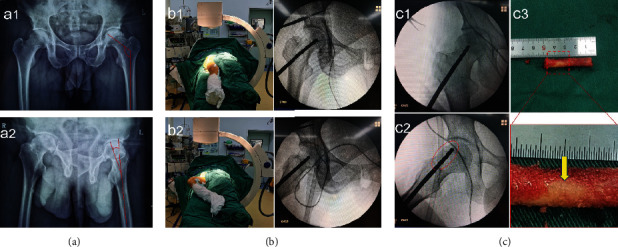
Clinical findings. (a) Conventional anteroposterior (a1) and lateral hip radiographs (a2) showing the neck shaft angle and anteversion angle. (b) Positioning of anteroposterior (b1) and frog position radiograph (b2) during core decompression. (c) Radiographs of injuring the cortical bone during surgery. (c1) Anteroposterior radiograph shows the good position of core decompression. (c2) Frog position radiograph shows the positioning to be slightly forward and does not exceed the bony margin of the femoral neck, while the cortical bone has been injured (red oval). (c3) Bone structure showing damaged femoral neck cortical bone (yellow arrow).
